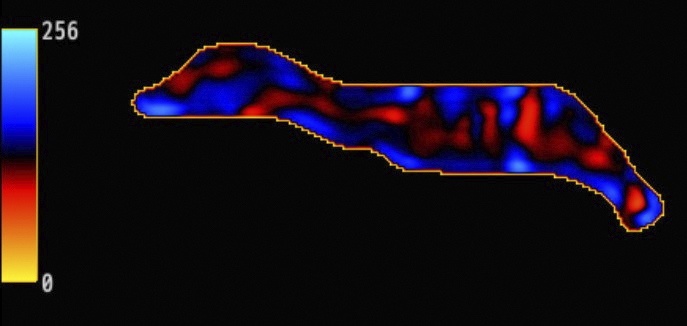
Magnetic resonance elastography (MRE) demonstrated that the mass was notably stiffer than the mammary gland (Figure 2). Areas marked with a crosshatch symbol (X) indicate regions unsuitable for stiffness measurement. Stiffness maps matching the anatomical structure of the contralateral breast were obtained, and waves propagated without interference in the MRE wave images (Figure 3). The breast magnetic resonance imaging (MRI) was obtained using a 3.0-T MRI system (Signa™ Pioneer; GE HealthCare, Milwaukee, WI, USA). Breast MRE imaging parameters are set as follows: a 16-channel breast coil, repetition time = 1000 ms, echo time = 59.2 ms, motion encoding gradient frequency = 80 Hz, vibration frequencies = 60 Hz, vibration stimulation = 70%, temporal phases = 4, number of excitations = 2, field of view = 380 mm, acquisition matrix = 64 × 64, slice thickness = 8 mm, number of slices = 13, pixel size = 5.9 × 5.9 × 8 mm^3^, and acquisition times of 69 s.
